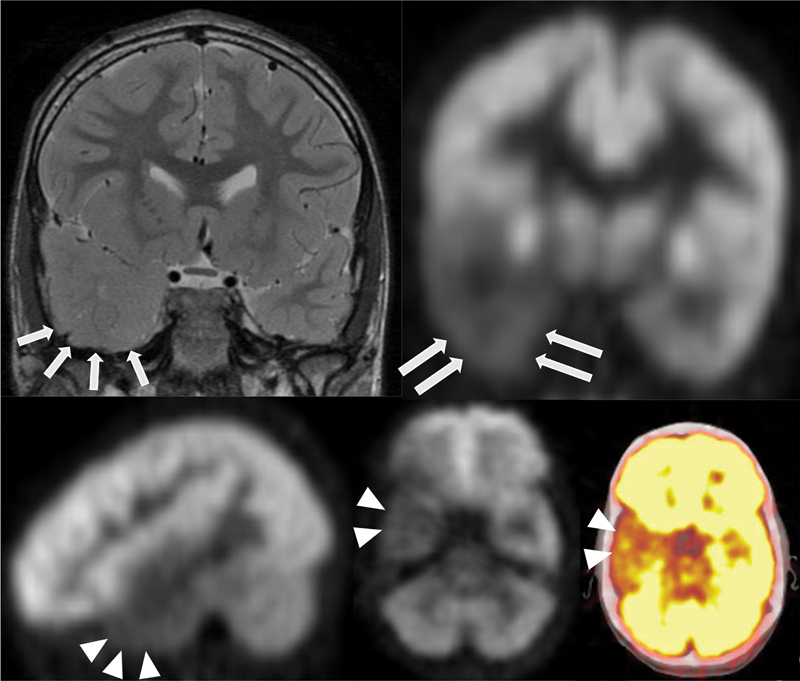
Brain MRI in patient with focal cortical dysplasia visualized as abnormal cortical thickening and effacement of white matter in the right temporal pole, best visualized on thin coronal T2-weighted MRI (
